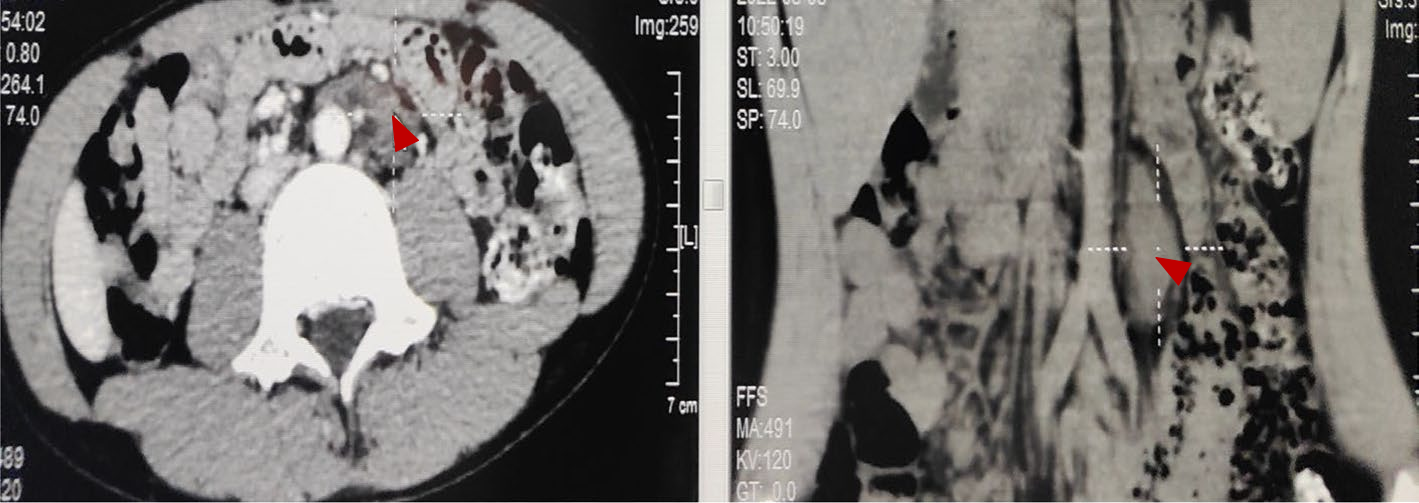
Abdominal CT images of the patient. Red arrows showed a soft tissue mass on the left side of the retroperitoneal abdominal aorta (3.8*2.0*1.8 cm)
